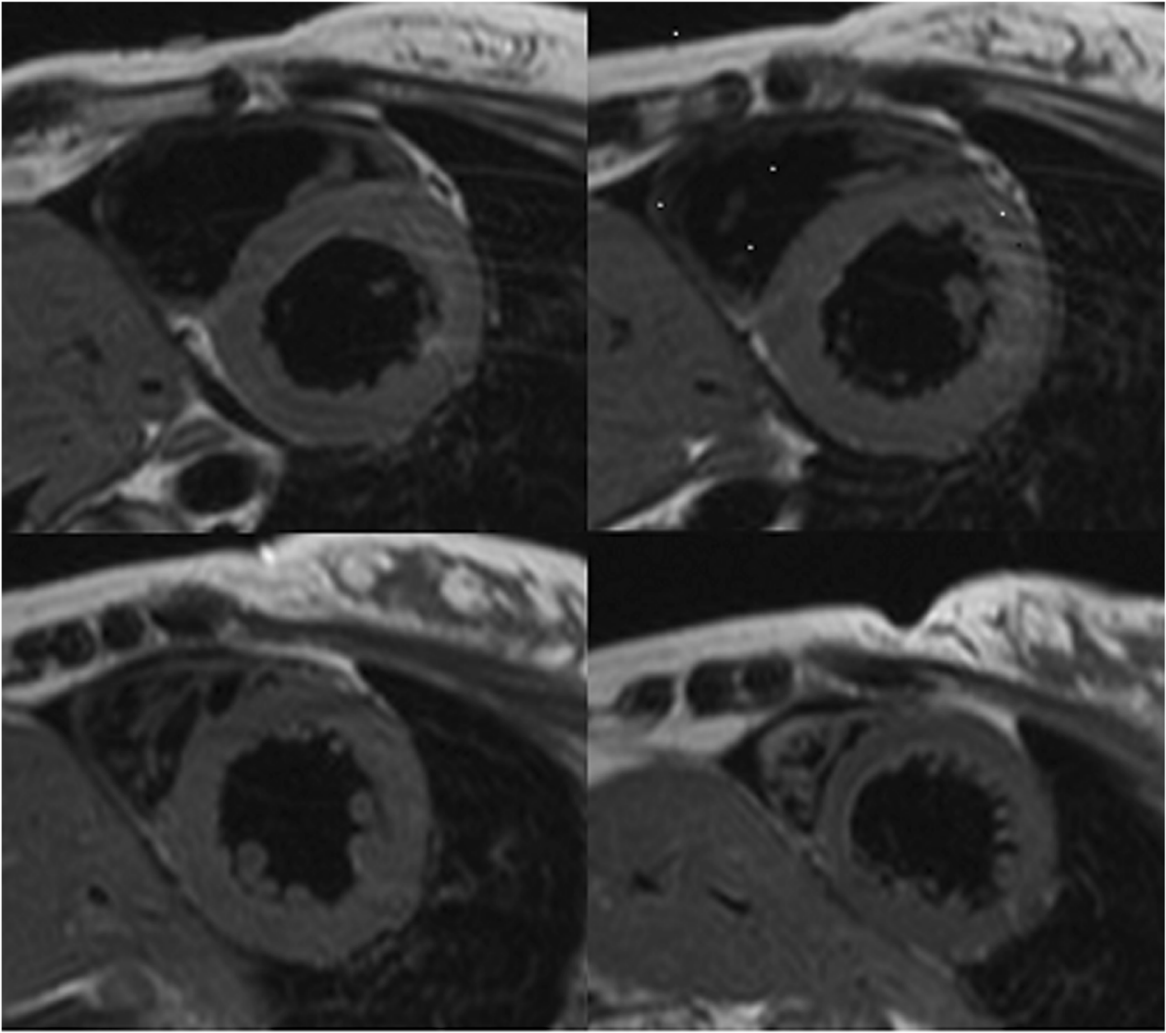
Short-axis, dark- blood T2- weighted cardiac magnetic resonance imaging demonstrating no signs of myocardial edema
